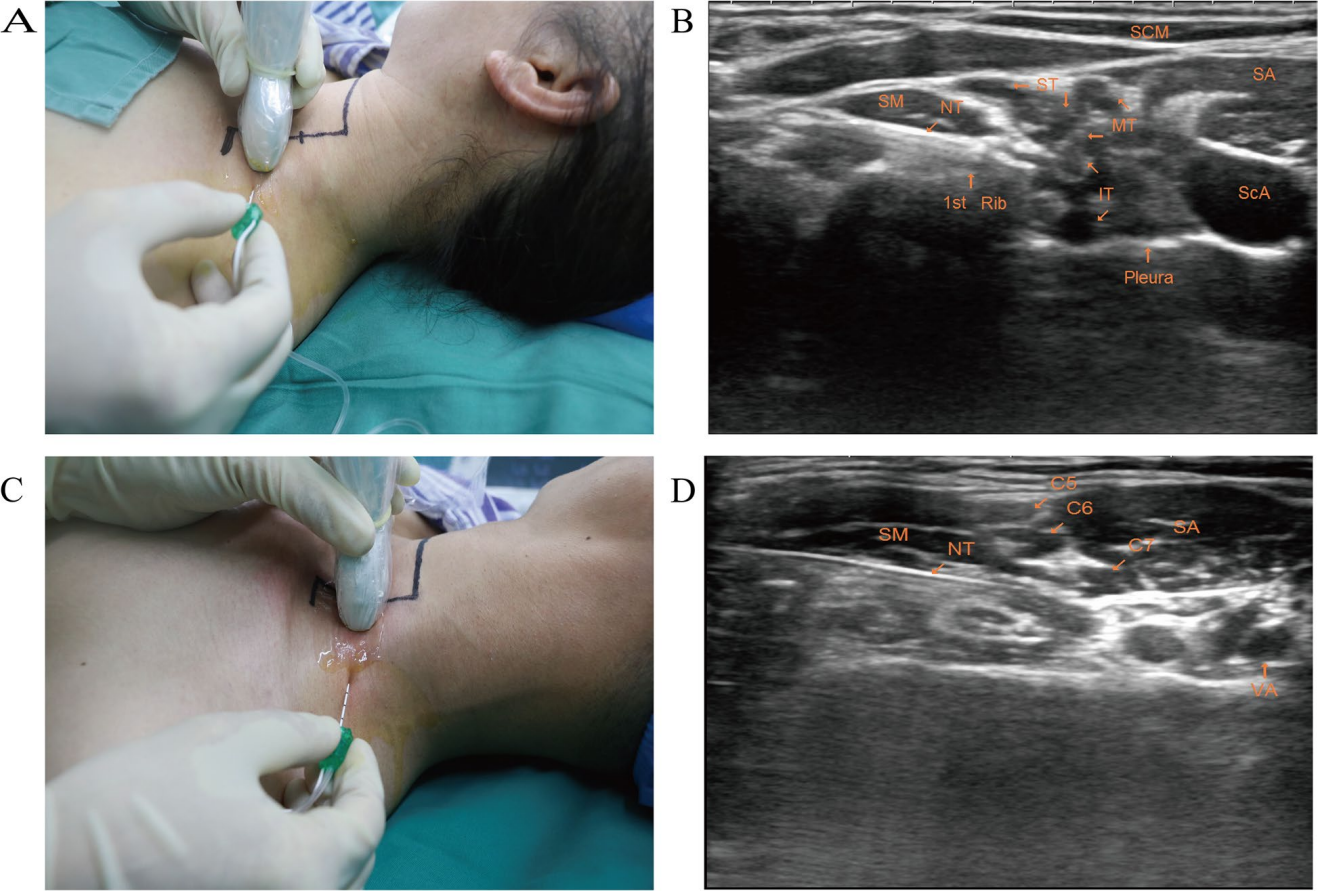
Location and an ultrasound image of LISB and MISB. Note: A and C: The patient in LISB group (A) or MISB group (C) was kept in the supine position with their heads facing away from the side of the block, and the transducer was placed on the interscalene groove, located 2/3 (A) or 1/2 (C) of the caudal distance from C6 to the clavicle. B and D: Ultrasonic image of LISB (B) or MISB (D) shows the first needle trajectory in-plane and advanced toward the lower part of the brachial plexus. I.T. = inferior trunk; MISB = middle interscalene brachial plexus block; M.T., middle trunk; LISB = low interscalene brachial plexus block; N.T., needle trajectory; S.A., scalenus anterior muscle; ScA, subclavian artery; SCM, sternocleidomastoid muscle; S.M., scalenus medius muscle; S.T., superior trunk; V.A., vertebral artery
